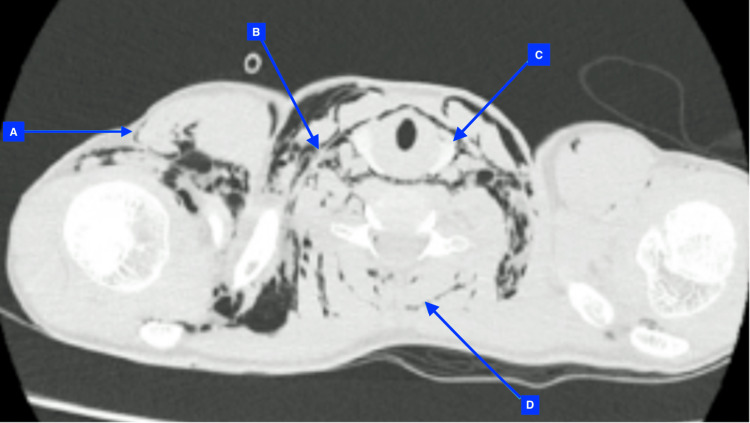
HRCT axial sections through upper chest and neck Note subcutaneous air (A), intermuscular air (B), paratracheal air (C) and air in between erector spinae muscles (D)
